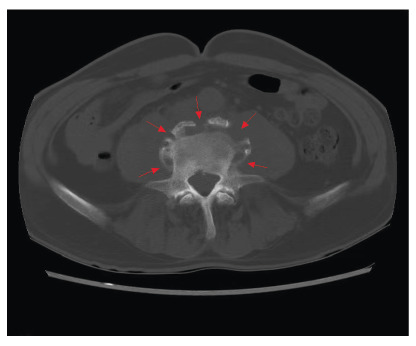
Computed tomography scan (transverse section) showing apparent destruction around the edge of the L4 vertebral body with prominent osteosclerosis and osteophytes (red arrows) resembling “petal rims”.
